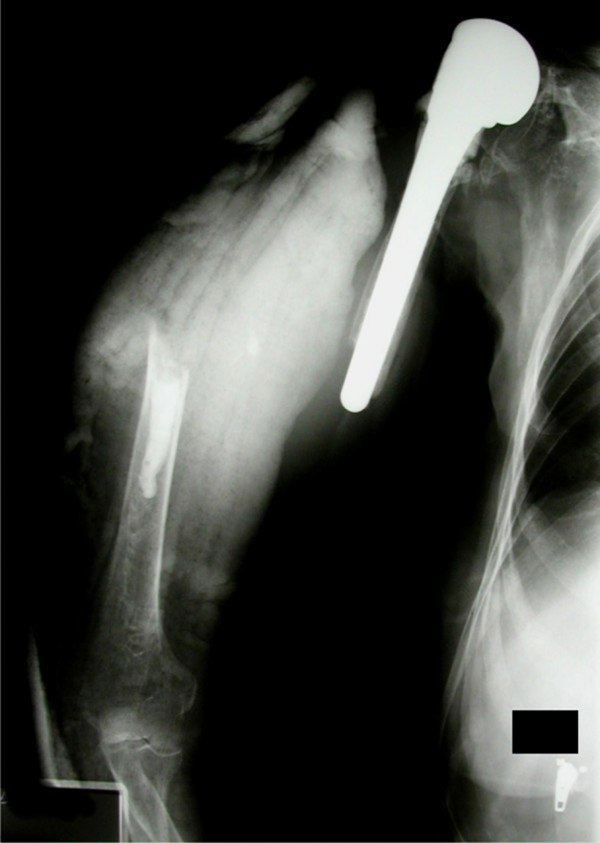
Plain radiograph showing gross displacement at the fracture site.
